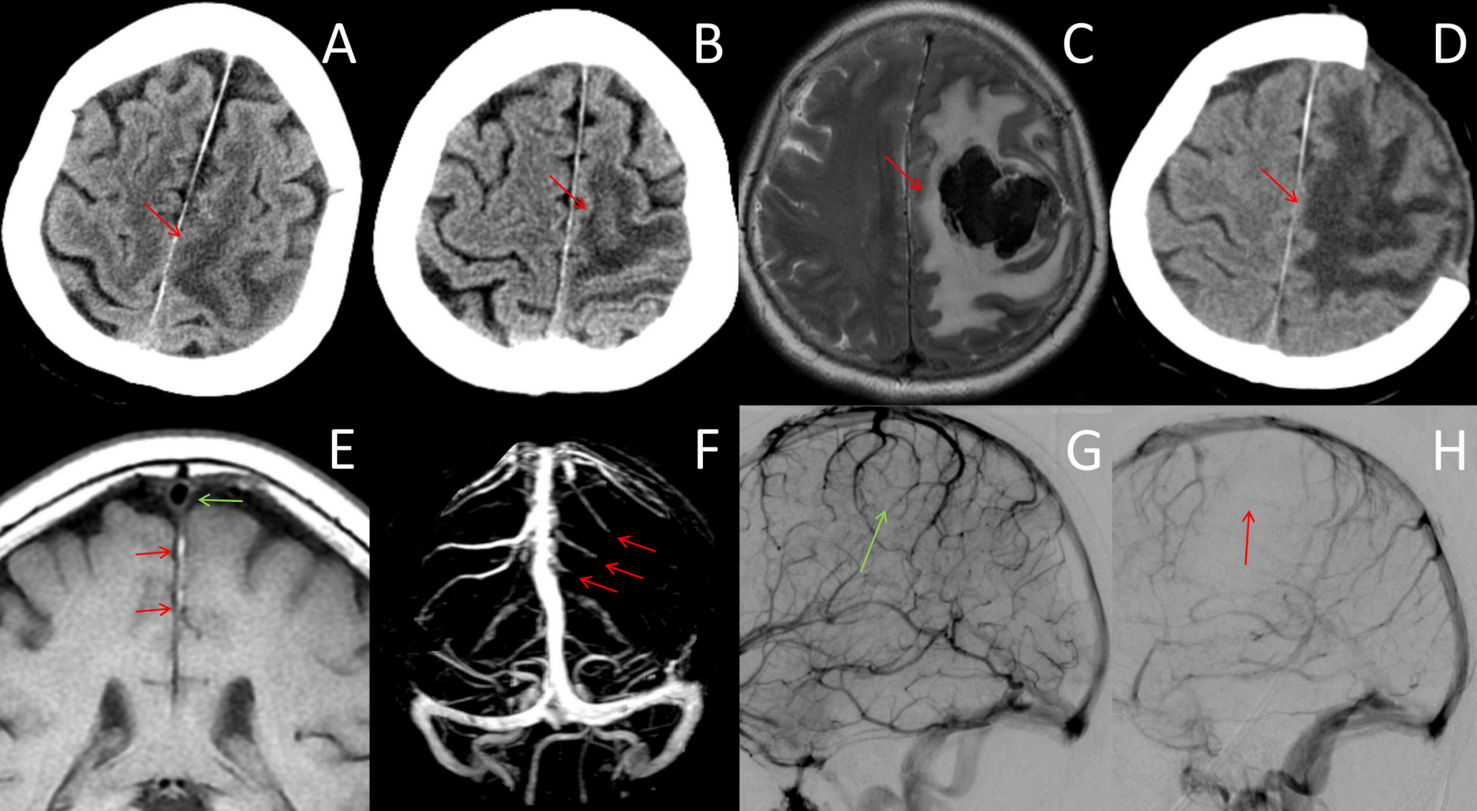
Neuroradiological data after day 4. (A, B) CT images on days 6 and 10 showed progressive enlargement of the venous infarction lesion in the frontoparietal cortex (red arrows). (C) MRI on day 12 showed venous infarction lesions with a large acute hematoma in the left frontoparietal lobe, which resulted in transtentorial herniation (red arrows). (D) CT scan after decompression craniectomy showed large areas of low-density lesions (red arrow). (E) MR black-blood-thrombus imaging (MRBTI) on day 7 showed vein thrombosis that extended proximally to the superior sagittal sinus (red arrow). The superior sagittal sinus was free of thrombosis (green arrow). (F) MR venography showed filling defects of the left frontal and partial cortical veins (red arrows). (G, H) DSA on day 8 showed normal venous flow of the right hemisphere (G, green arrow) compared with multiple thromboses in the left superior and internal cerebral veins of the left hemisphere (H, red arrows).
